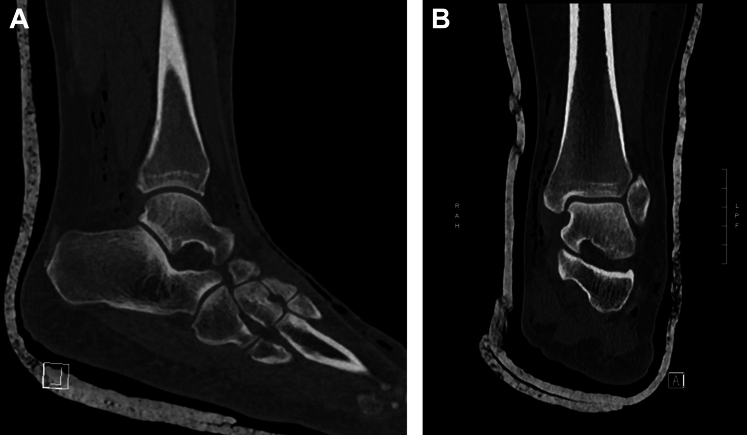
(A and B) Postreduction computed tomography with gas tracking in subcutaneous space.
